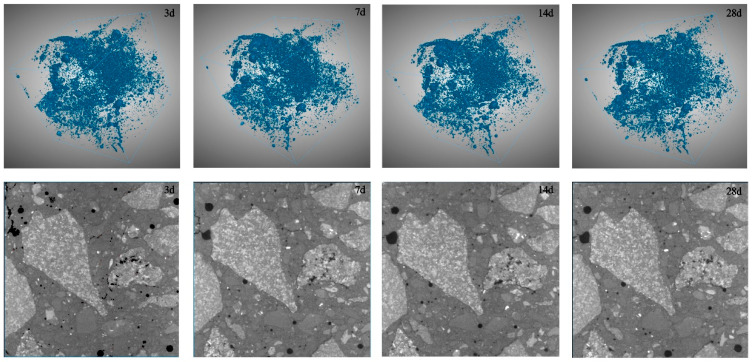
X-CT Image of SCC with 4% CAI dosage.
